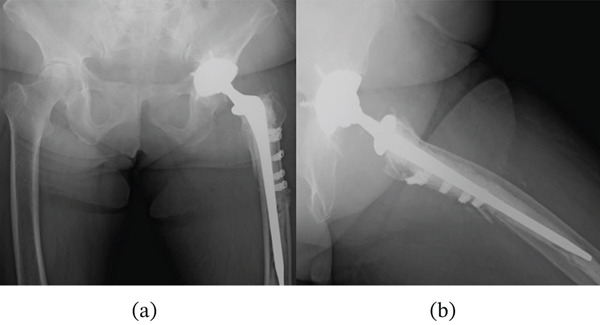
Postoperative radiographs after revision THA: (a) anteroposterior view and (b) lateral view.
